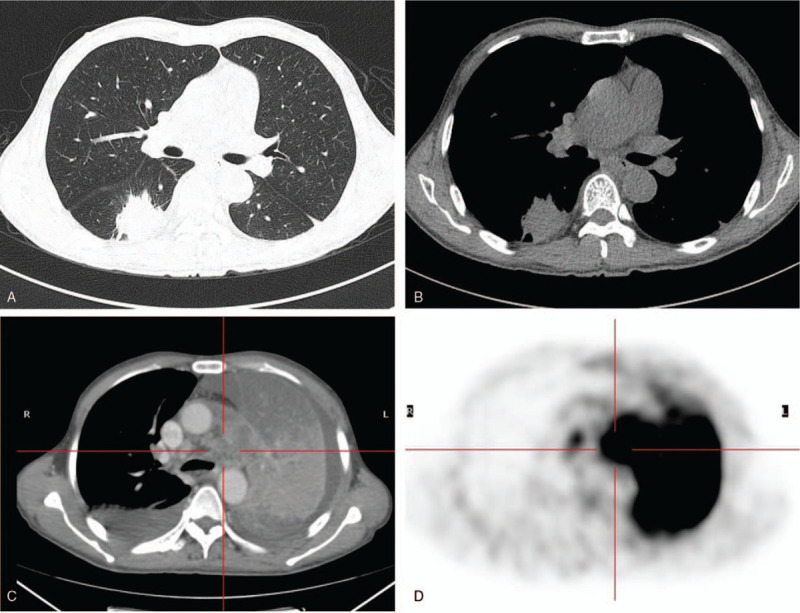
Male, 59 years old, repeated chest pain in a month and a massive opacity in the right lung for 3 weeks. CT shows a massive opacity with lobulation and speculation in the margin of the lesion, which has a uniform density and mild-moderate enhancement in the superior segment of the right lower lung (A and B). Four months later, the left lung shows large atelectasis with bilateral pleural effusion (C). PET/CT shows atelectasis in the left lung with a high concentration of radioactivity in the maximum SUV value of 19.9 (D).
